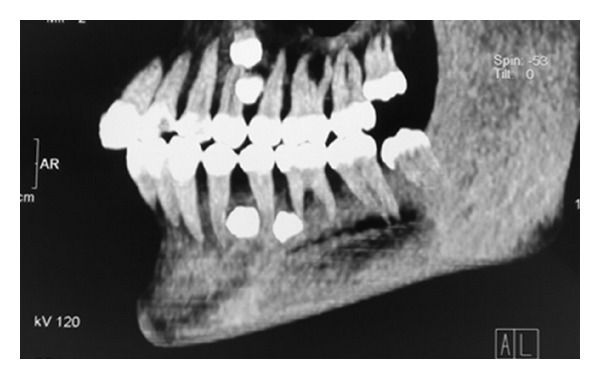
CT scan view of the left side to locate exact position of four supernumeraries.
